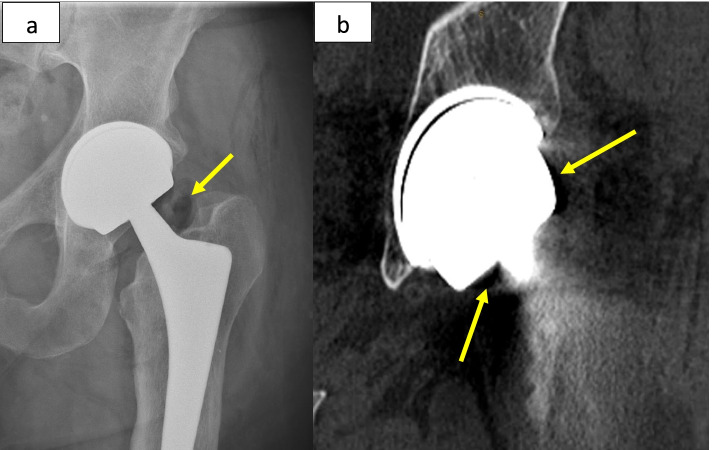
a Anteroposterior left hip revealing a thin crescent shaped radiolucency between the acetabular shell and the ceramic liner and pneumarthrosis (arrow). b CT-scan view of the left hip demonstrating the thin crescent shaped radiolucency between the acetabular shell and the ceramic liner, 2 arrows are pointing at the intra-articular gas
